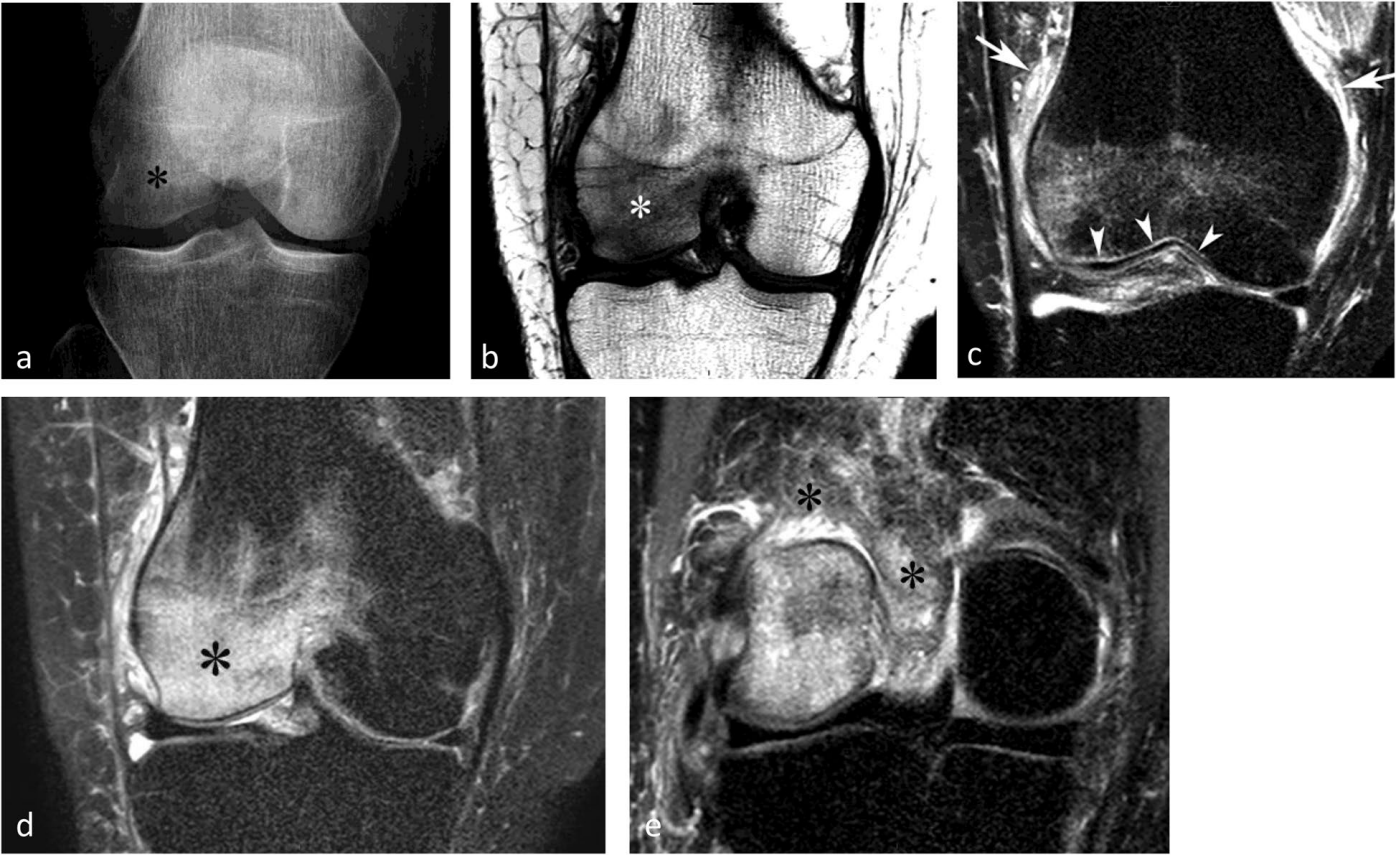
Typical pattern of complex regional pain syndrome type I (CRPS1). a Radiograph shows intense bone loss in the lower pole of a condyle (asterisk). b–e T1-weighted (b) and fat-suppressed T2-weighted (c–e) MR images show a BME-like pattern similar to that of a SIF (asterisks in b and c). Note the particularly intense edema-like signal intensity also in the soft tissues (arrows in c and asterisk in e), as well as a very fine linear area with intense high signal intensity, immediately adjacent to the subchondral bone plate (arrowheads in c)
